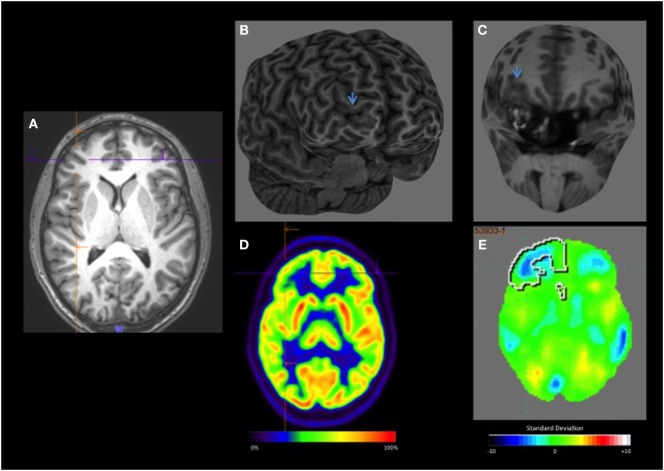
(A) Axial T1 negative-MRI (B,C) curvilinear reconstruction shows better the cortical thickening and abnormalities of sulcus and gyrus pattern in the right anterior frontal lobe. (D) visual-positron emission tomography (PET) showing slight hypometabolism in the right frontal lobe. (E) Quantitative-PET shows hypometabolism in the right frontal lobe −3.7 SD.
